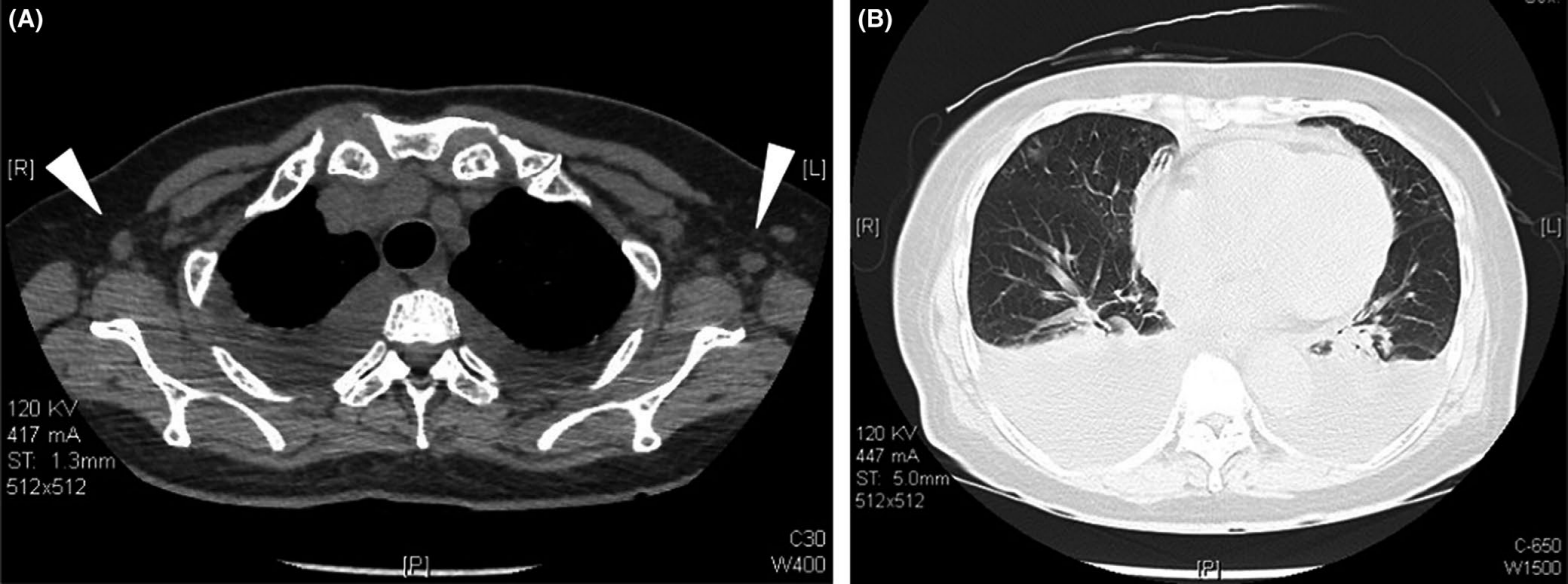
Chest computed tomography shows enlarged lymph nodes at the bilateral axillary area (white arrow heads) (A) and pleural effusion (B)
